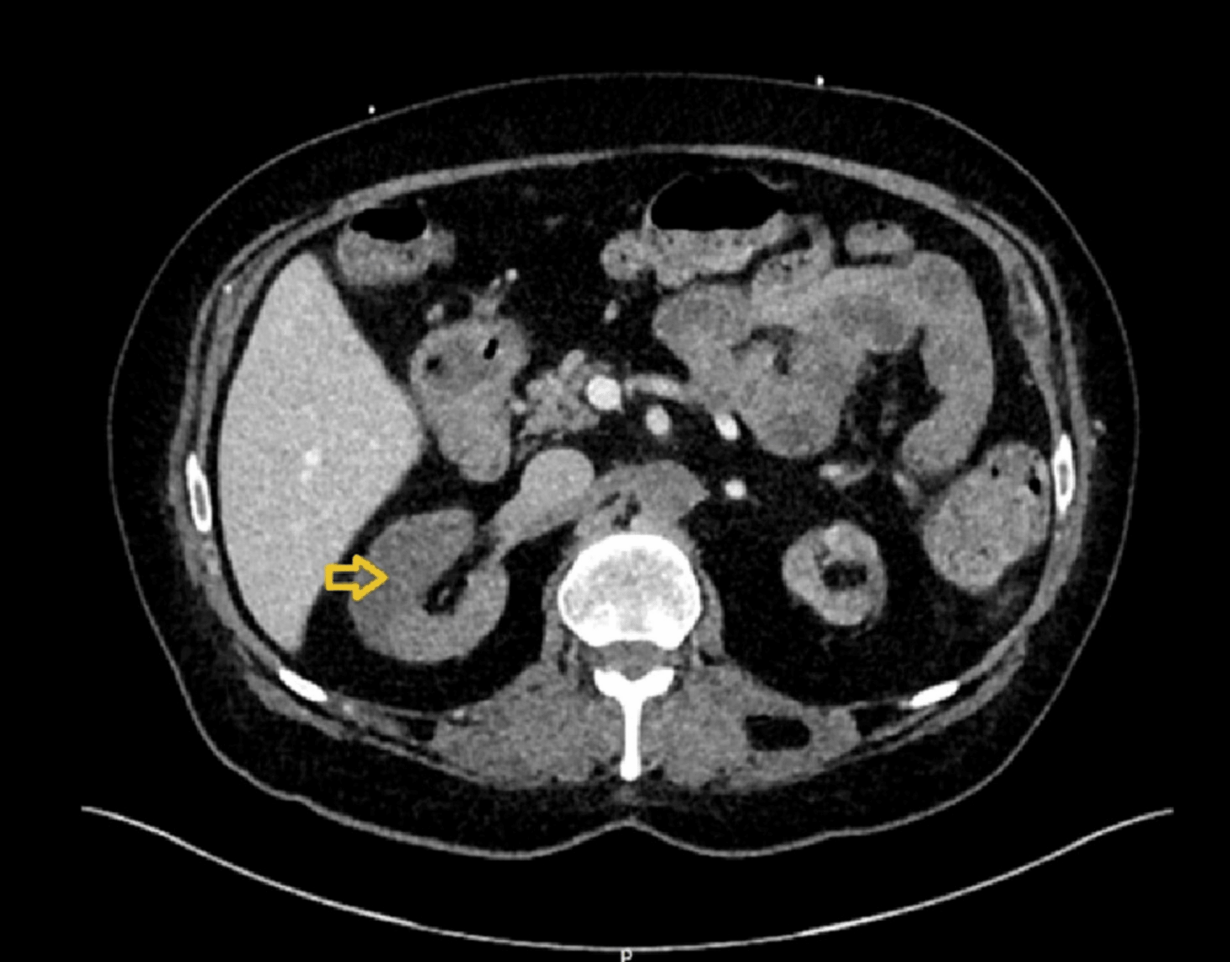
Right kidney infarct The above CT scan showed an acute infarction (yellow arrow) in the right kidney due to renal arterial occlusion.
